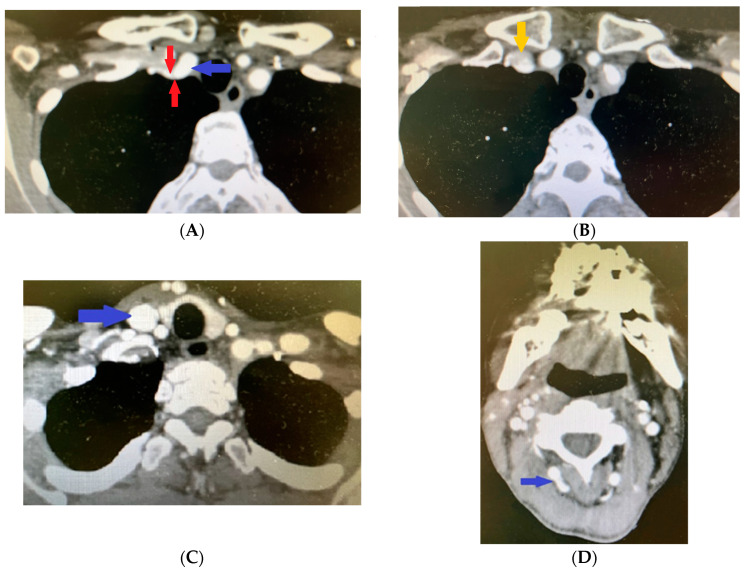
Contrast-enhanced computed tomography revealing (A) dilated right subclavian artery (blue arrow) followed by significant stenosis (red arrows); (B) parietal thrombosis in the right subclavian artery (yellow arrow); (C) aneurismal dilatation of the right external carotid artery (blue arrow); (D) fusiform dilatation of the right vertebral artery (blue arrow).
